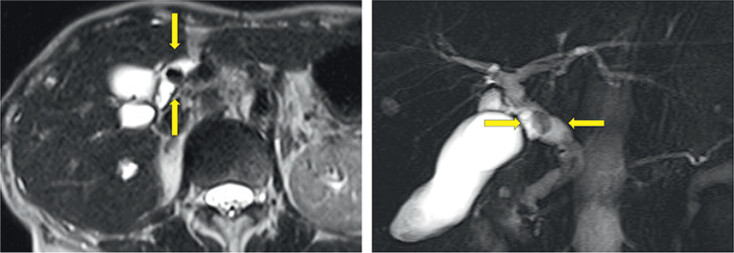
In an axial view, T2-weighted magnetic resonance imaging showed
